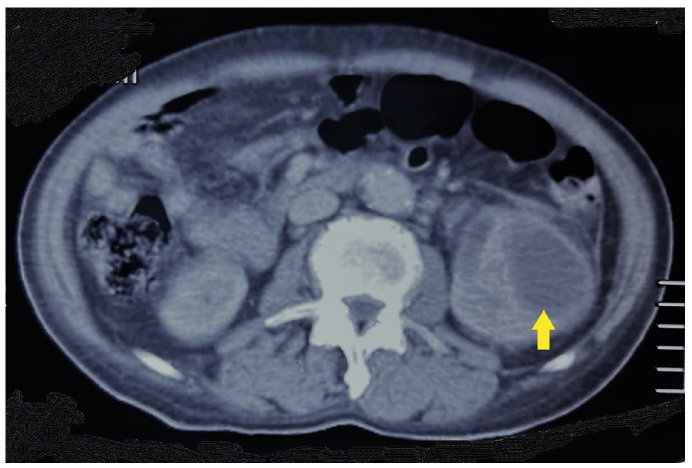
Computed tomography of the abdomen and pelvis showing well-defined collections in the left kidney resembling abscesses, with the largest collection measuring 3.7 cm × 3.8 cm × 3.9 cm at the lower pole (indicated by arrow).
